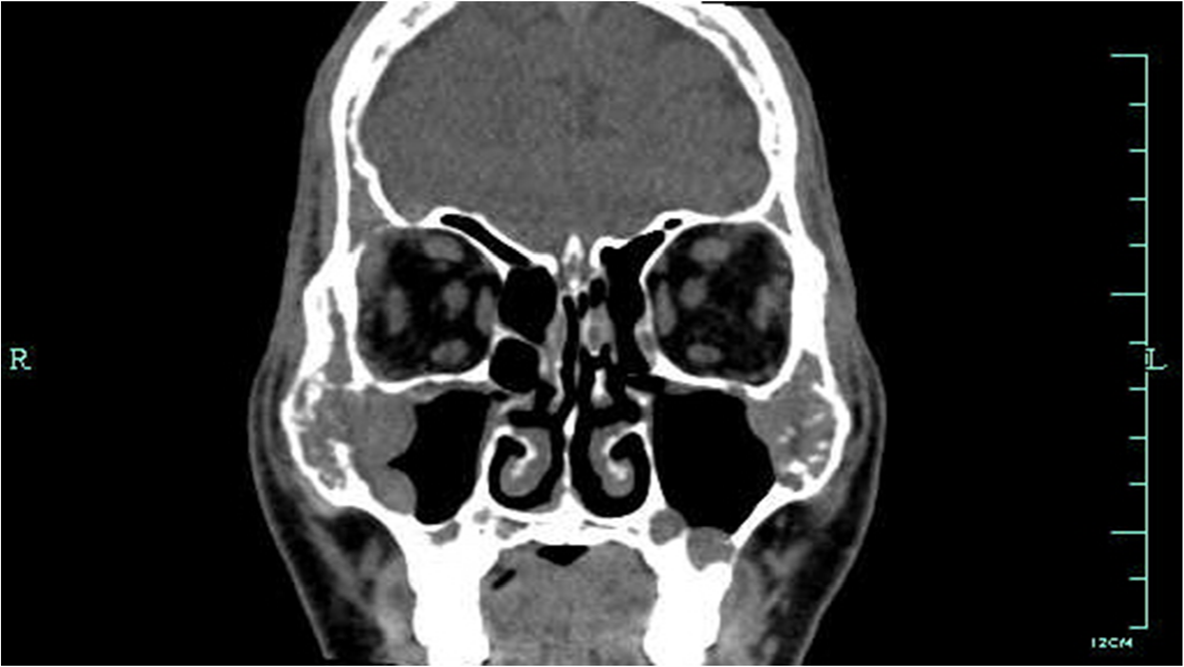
Computed tomography of the sinus spiral. Computed tomography of the sinus spiral showing a pansinusitis and diffuse bone destruction of craniofacial bone.
